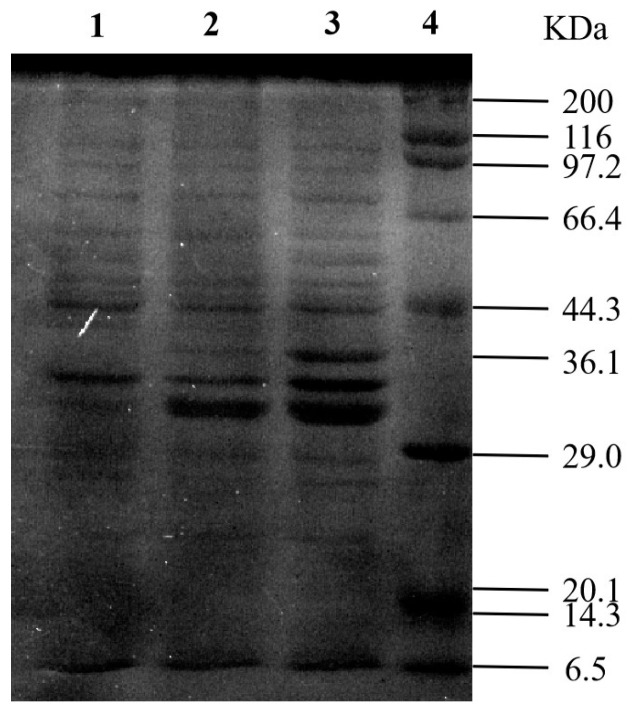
Analysis of feruloyl esterase (KoFae) by SDS-PAGE. Lane 1: IPTG-induced crude protein expressed by *E. coli* carrying plasmid pET-28a; lane 2: IPTG-induced ultrasound-treated supernatant of *E. coli* carrying plasmid pET-28a-KoFae; lane 3: I IPTG-induced crude protein expressed by *E. coli* carrying plasmid pET-28a-KoFae; lane 4: standard protein marker.
